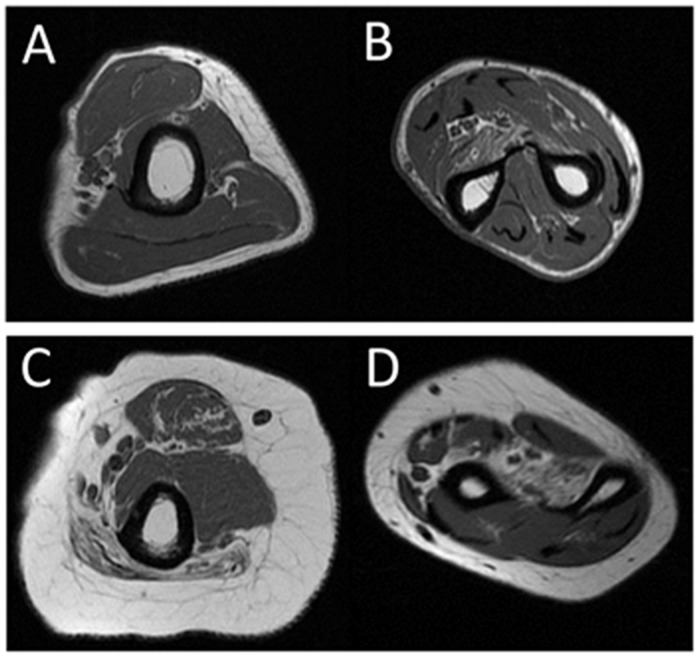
Muscle MRI findings of the upper limb muscles in myotonic dystrophy type 1. Typical findings of muscle MRI in a patient with mild disease, patient 4 (A, B), and a patient with severe disease, patient 7 (C, D), as summarized in Table 1. On T1-weighted images, only the FDP muscle showed high intensity signals with fatty degeneration in patient 4. In patient 7, the FDP muscle as well as a few other muscles showed high intensity signals with fatty degeneration. A, C = arm muscles; B, D = forearm muscles.
